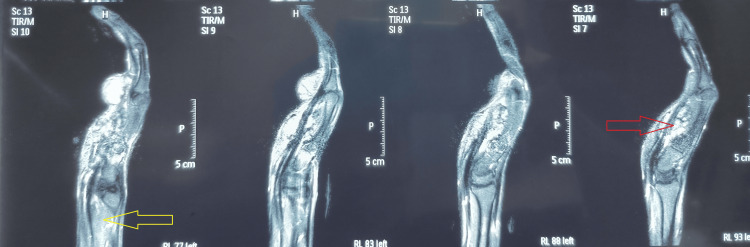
MRI right hand T1-weighted imaging post contrast MRI sagittal view showed the extent of the multilobulated giant cell tumor flexor tendon sheath, invading the mid-carpal space (as shown by the red arrow), crossing the wrist joint into the distal ulnar aspect of the forearm (as indicated by the yellow arrow)
